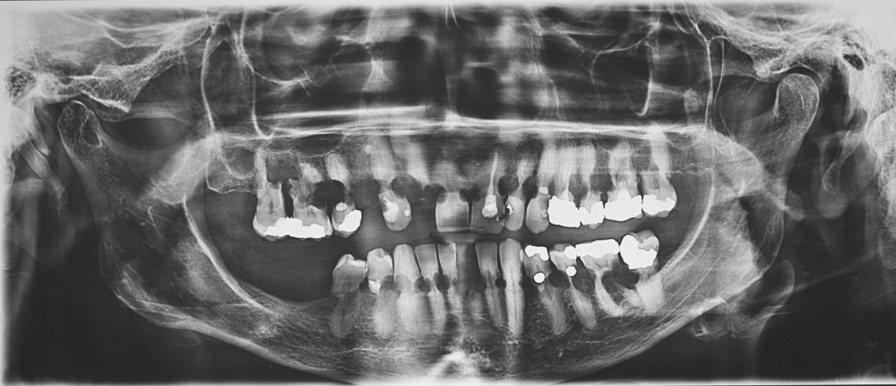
A panoramic radiograph taken in July 2020 showing generalized external cervical root resorption
